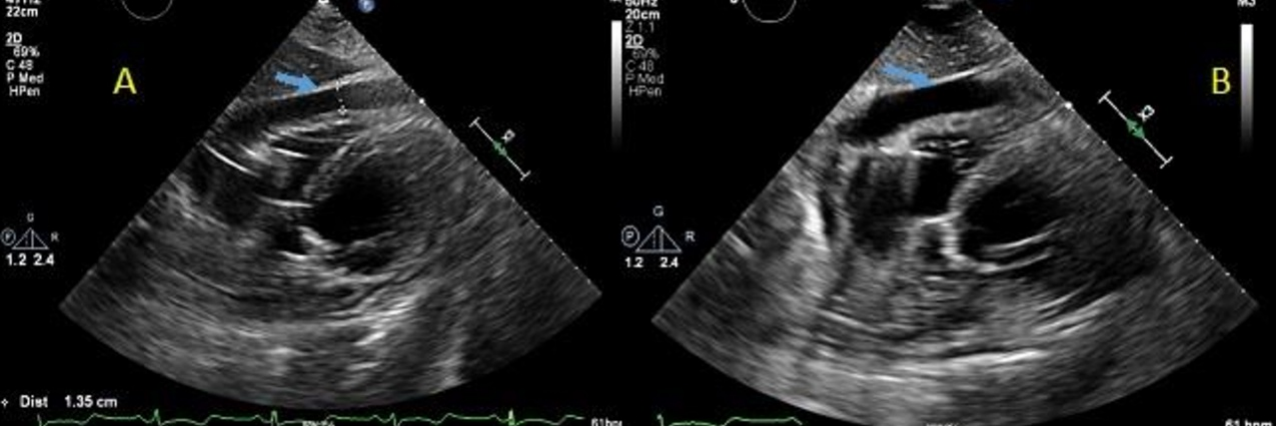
Echocardiogram showing pericardial effusion causing right atrial compression in diastole
